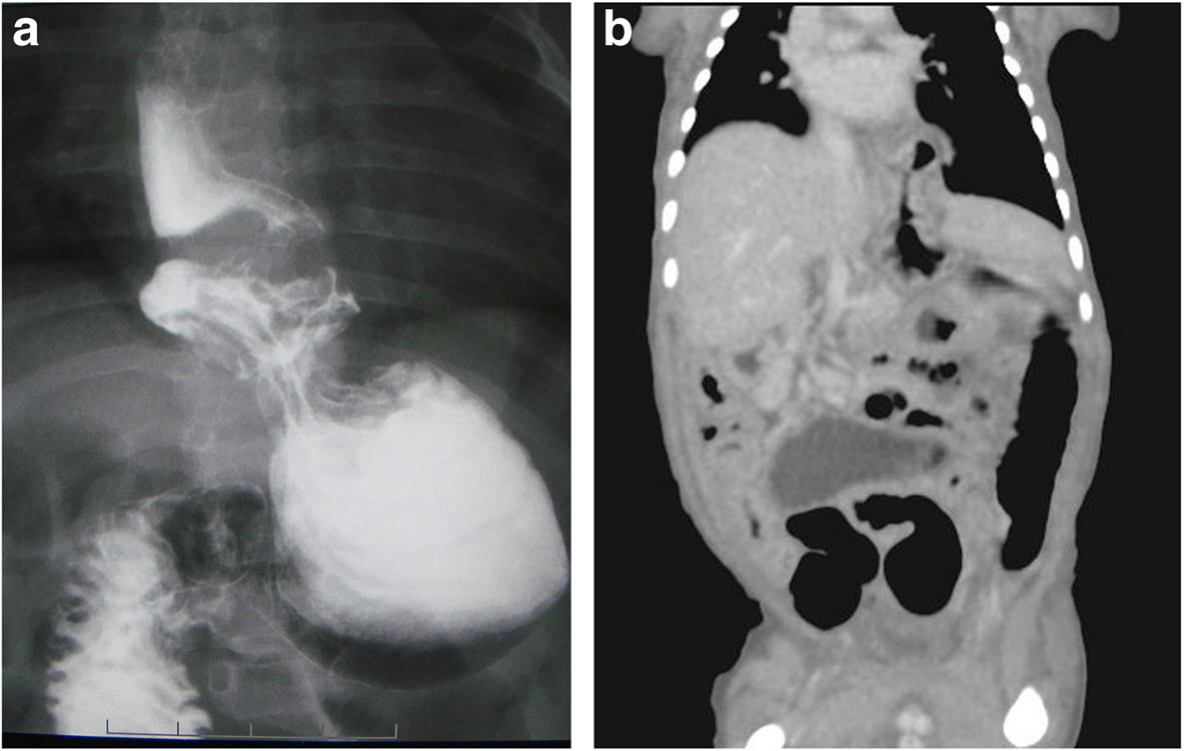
a Upper gastrointestinal series on the 51st day revealed a sliding type hiatal hernia and marked GER with crooked esophagus. b Coronal view of the enhanced CT scan on the 53rd day also showed sliding type hiatal hernia
